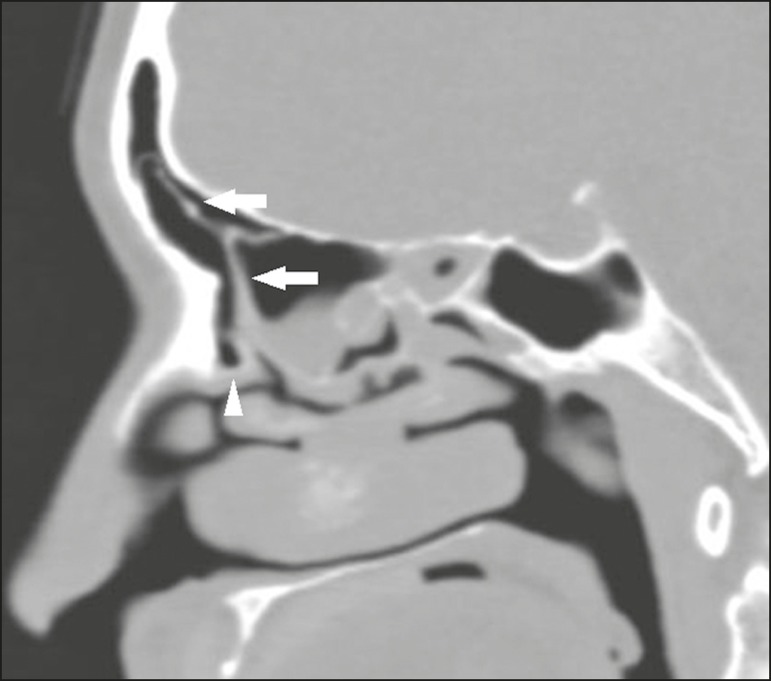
Sagittal CT slice, with bone window settings, showing a large
